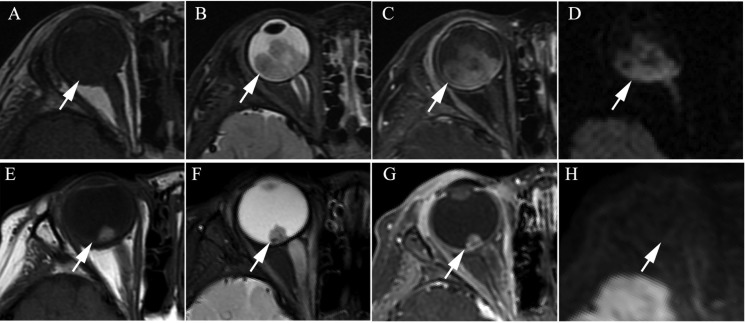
Clinically diagnosed retinoblastoma in a 24-month old male (patient 1) (A–H) An orbital mass is shown at the right globe with the tumor totally or partially covering the optic disk before intra-arterial chemotherapy (IAC), which demonstrates a slightly higher signal intensity than the ocular fluid as observed in axial T1-weighted magnetic resonance imaging (MRI) (A), low signal intensity (arrow) in the axial T2-weighted MRI (B) and moderate enhancement of the retinoblastoma (arrow) in the axial contrast-enhanced, T1-weighted, fat-saturated MRI (C). The axial DWI (D) shows restricted diffusion (arrow) in the tumor, a finding indicative of high tumor cellularity. (E–H) The axial T2-weighted MRI (F) shows that the size of the tumor is diminished after treatment with IAC and demonstrates a low signal intensity of the residual tumor foci partially covering the optic disk (arrow). The axial contrast-enhanced T1-weighted, fat-saturated MRI (G) shows non-enhancement of the residual tumor foci with comparison to the high signal intensity found in the T1-weighted MRI (E). The axial DWI (H) shows the low signal intensity of the residual tumor foci (arrow).
